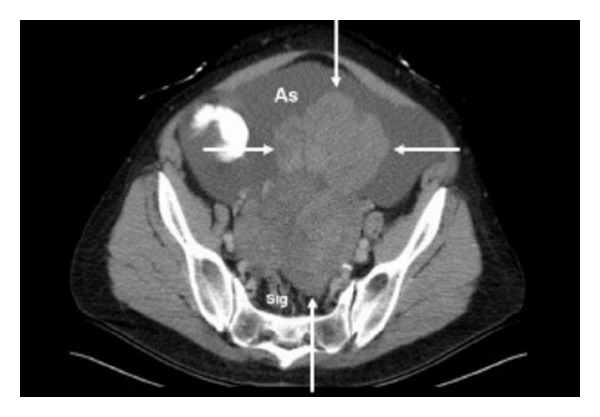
CT axial image of lower abdomen/pelvis demonstrating large predominantly solid mass (arrows) that appears to be arising from the retroperitoneum, surrounding sigmoid colon (Sig) and extending into peritoneal cavity. Mass is surrounded by a large amount of ascites (As).
